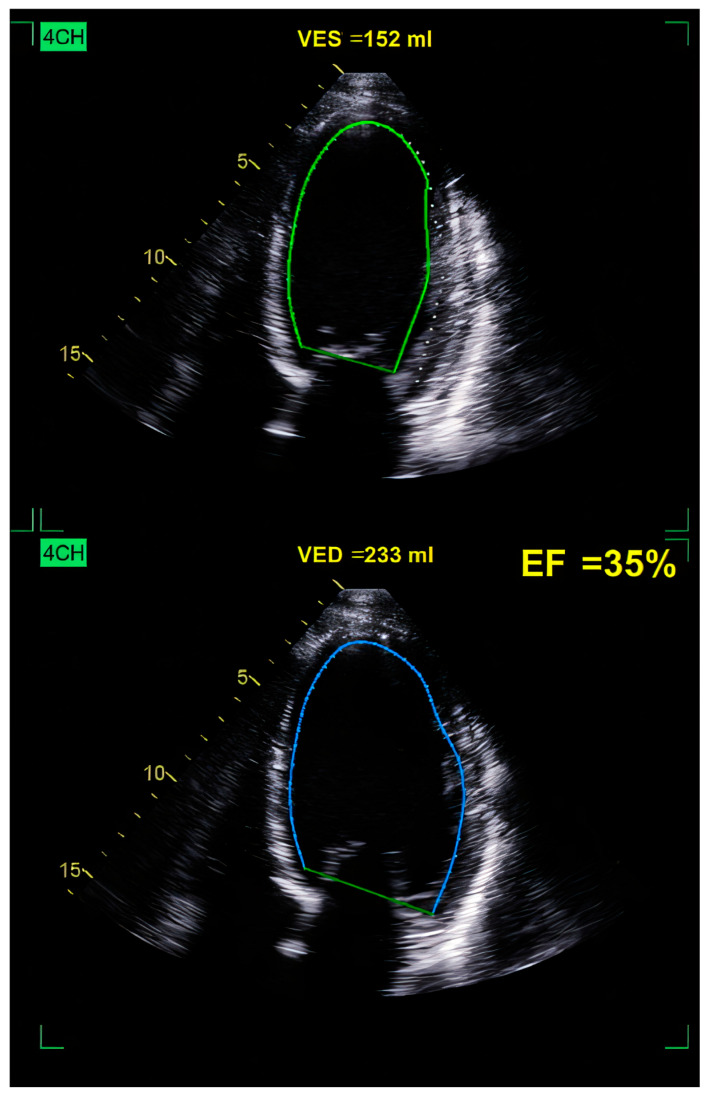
Two-dimensional TTE showing a dilated LV, with high VED of 233 mL, marked by the blue contour and increased VES of 152 ml emphasized by the green contour. Global LV hypokinesia and reduced EF (ejection fraction) of 35% in a patient with ethanolic DCM. VES = end-systolic volume of LV, VED = end-diastolic volume of LV, 4CH = apical 4-chamber view.
